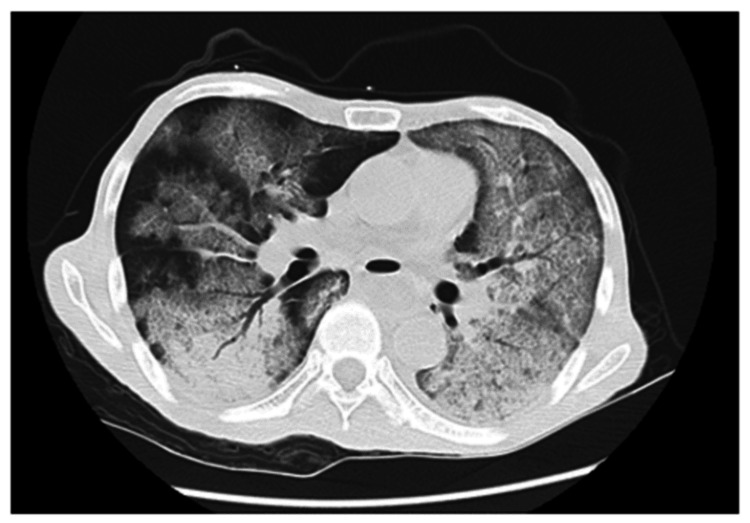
Chest CT Chest CT imaging revealing bilateral infiltrations CT: computed tomography
